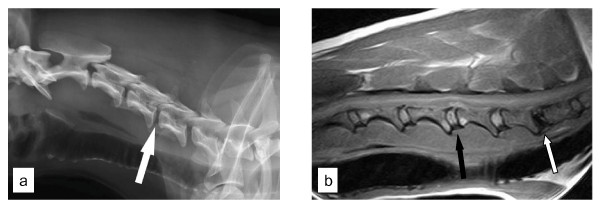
a. The radiographic examination of the cervical spine in a Dachshund with a surgically confirmed disc extrusion at C4-C5 (white arrow). Notice the absence of CDVR at C4-C5. b. A T1-weighted sagittal magnetic resonance image of the cervical spinal cord of the Dachshund in 3a. Notice the extent of calcification of the C6-C7 disc (white arrow) compared to the affected disc at C4-C5 (black arrow).
